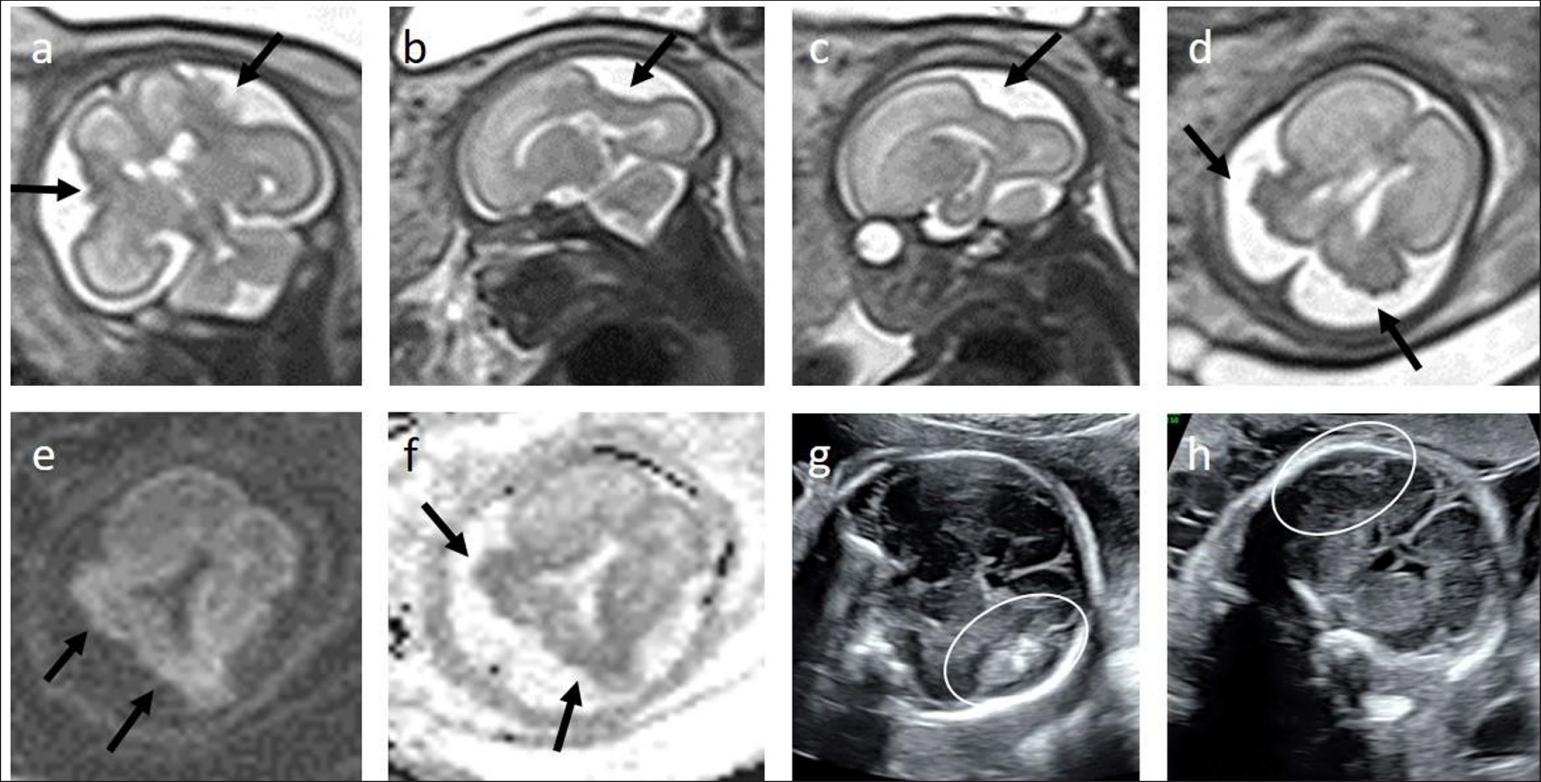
T2-weighted MRI images (a–d) and DWI (e) with corresponding ADC map (f) of an ex-donor at a gestation of 28 weeks (w) in a monochorionic diamniotic pregnancy complicated by TTTS with laser intervention at 20 w (Case 5 in Table 1). Prior US at 24 w (g) and 26 w (h) are also shown. Fetal MRI was performed for further evaluation of suspected subdural bleeding on the right, which appeared to remain stable in extension but with decreasing echogenicity (white circle in g and h). In addition, the fetus was also monitored closely with US for necrotizing enterocolitis with bowel distension and complicated ascites with peritoneal hyperechoic nodules (not shown). MRI at 28 w (a–f) demonstrates symmetrical bilateral cortical atrophy with irregular lining and hypo-intense signal on T2 (black arrow in a-d), in keeping with old ischemia. On b-1000 of DWI (e) and ADC (f) no acute ischemia was seen; only the same atrophy (black arrow in e and f) was evident.
